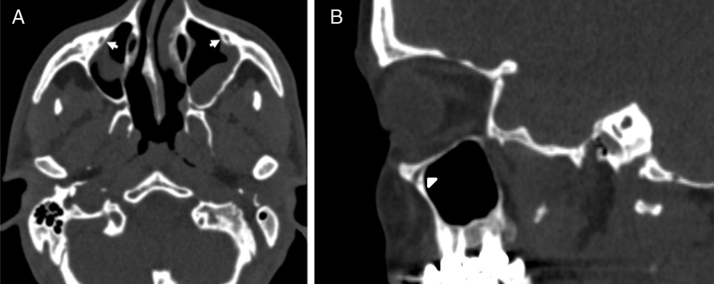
(A) Axial paranasal sinus CT image showing right-sided infraorbital canal Type 1 within the maxillary bony roof and left-sided infraorbital canal Type 2 partially protruding into maxillary sinus (thick arrows). (B) Right parasagittal image showing infraorbital canal Type 1 (arrowhead).IOC Type 2, partially protruding into the maxillary sinus (Fig. 2A and B).
